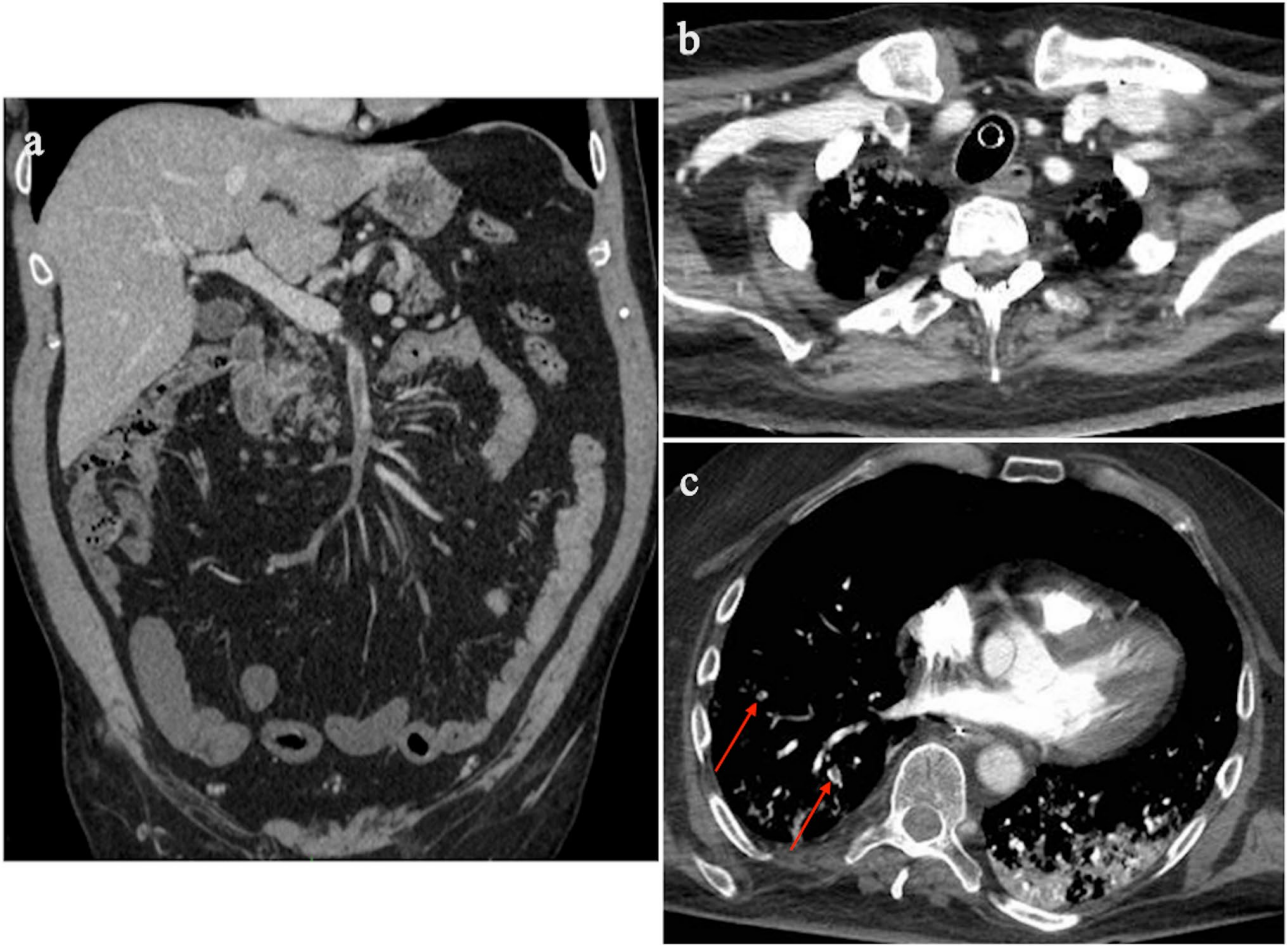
Thromboembolic complications detected incidentally on CT examinations of COVID-19 patients treated with VV-ECMO. a Coronal reformatted CT image of a patient with VV-ECMO and thrombosis of the superior mesenteric vein extending to several jejuno-ileal branches. b Axial CT image of a patient with thrombosis of the right internal jugular vein, in whom VV-ECMO had been removed two days earlier. c CT pulmonary angiography examination showing pulmonary embolism (arrows) at the posterior and anterior segments of the right lower lobe
